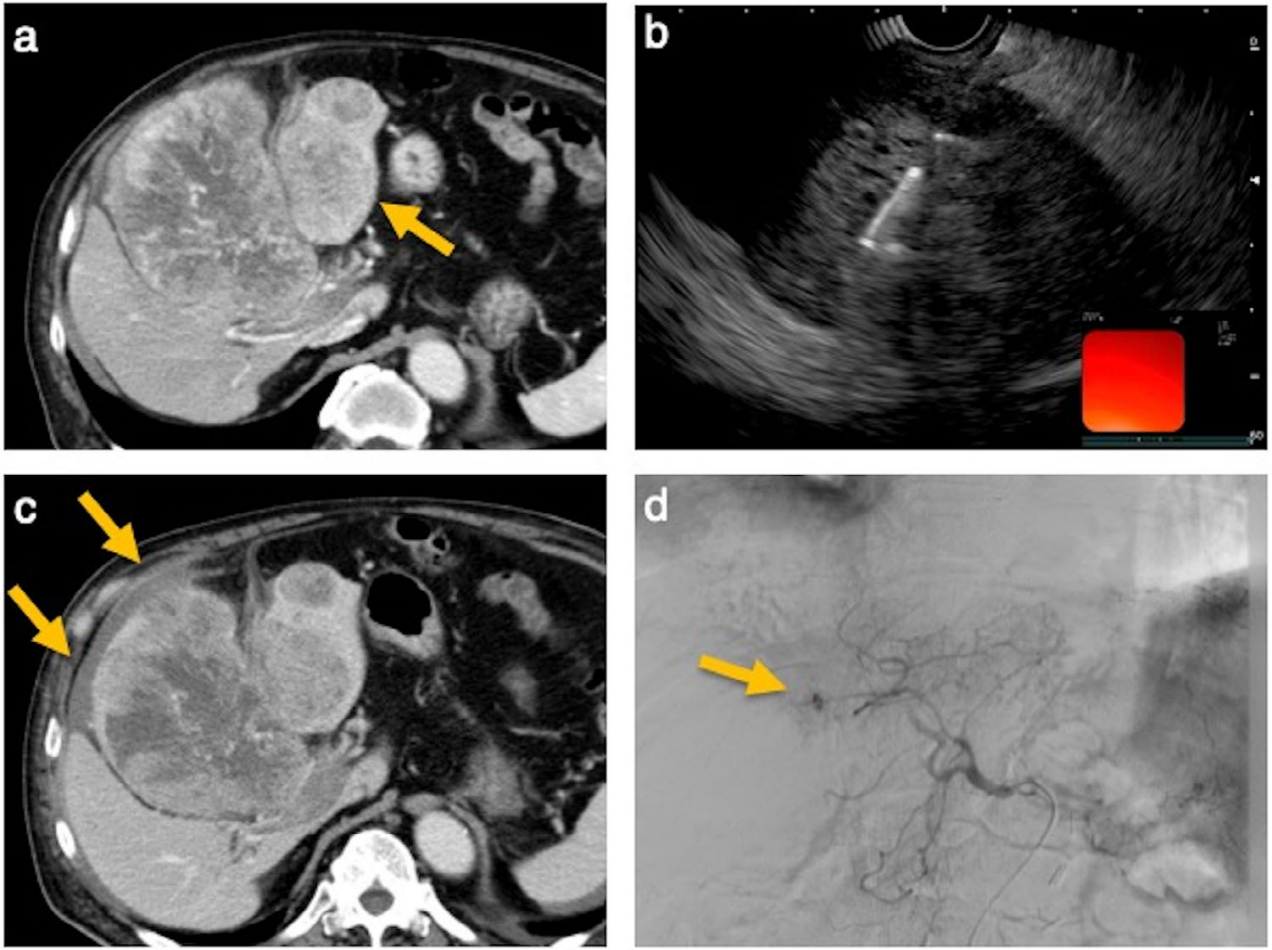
Post‐procedural bleeding following endoscopic ultrasound‐guided tissue acquisition (EUS‐TA). (a) Computed tomography (CT) image. A large mass is seen occupying the left hepatic lobe. The lesion in segment 2 is located adjacent to the stomach. (b) EUS‐TA was performed targeting the lesion in segment 2. (c) CT image 4 days after EUS‐TA. A fluid collection with higher attenuation than water is noted on the liver surface. Based on the clinical course, it was diagnosed as post‐procedural bleeding. (d) Angiographic image. Contrast extravasation from segment 4 was observed, and embolization was performed.
